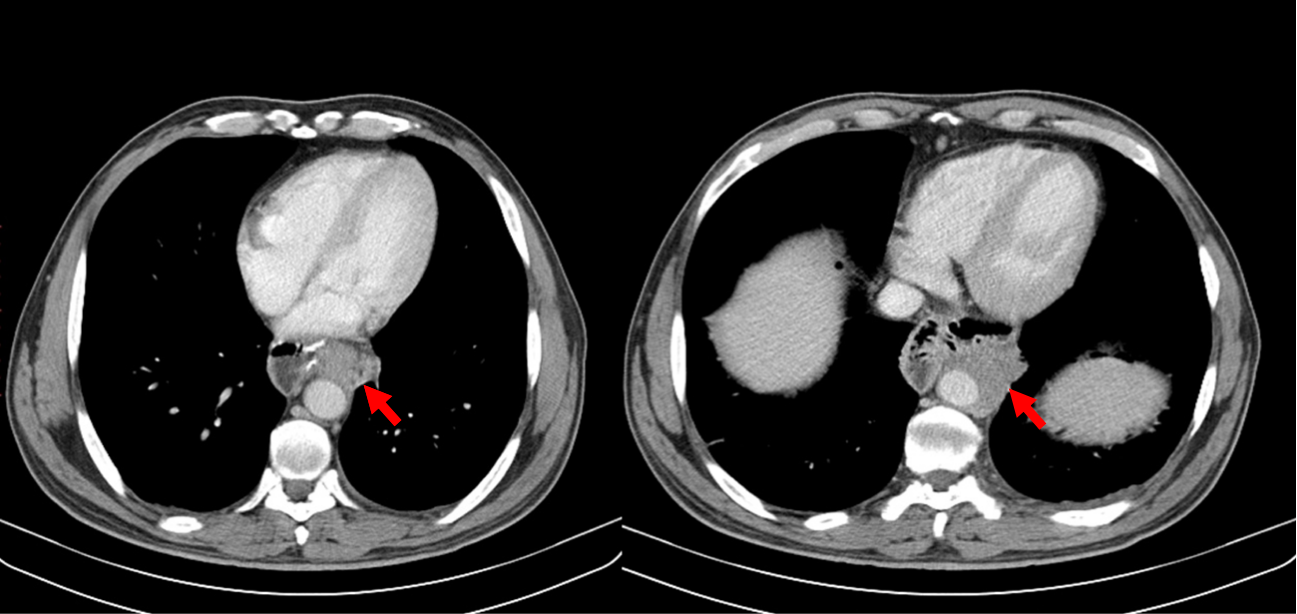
Thoracic-abdominal CT of a patient who had undergone total gastrectomy for adenocarcinoma some years earlier. There is evidence of a soft tissue mass next to the surgical anastomosis, encircling the descending aorta (*arrows*) and corresponding to a non-resectable neoplastic recurrence
